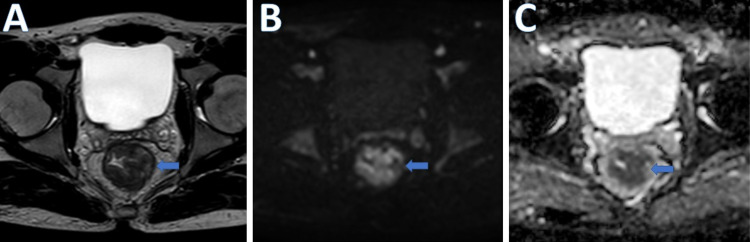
Pre-CRT images A. T2W MR axial image. B. DWI. C. ADC image of a 35-year-old man with pathologically proven moderately differentiated adenocarcinoma of the mid- and lower rectum (T3N1M0) showing circumferential tumor with diffusion restriction (blue arrows) CRT: chemoradiotherapy; DWI: diffusion-weighted imaging; ADC: apparent diffusion coefficient
